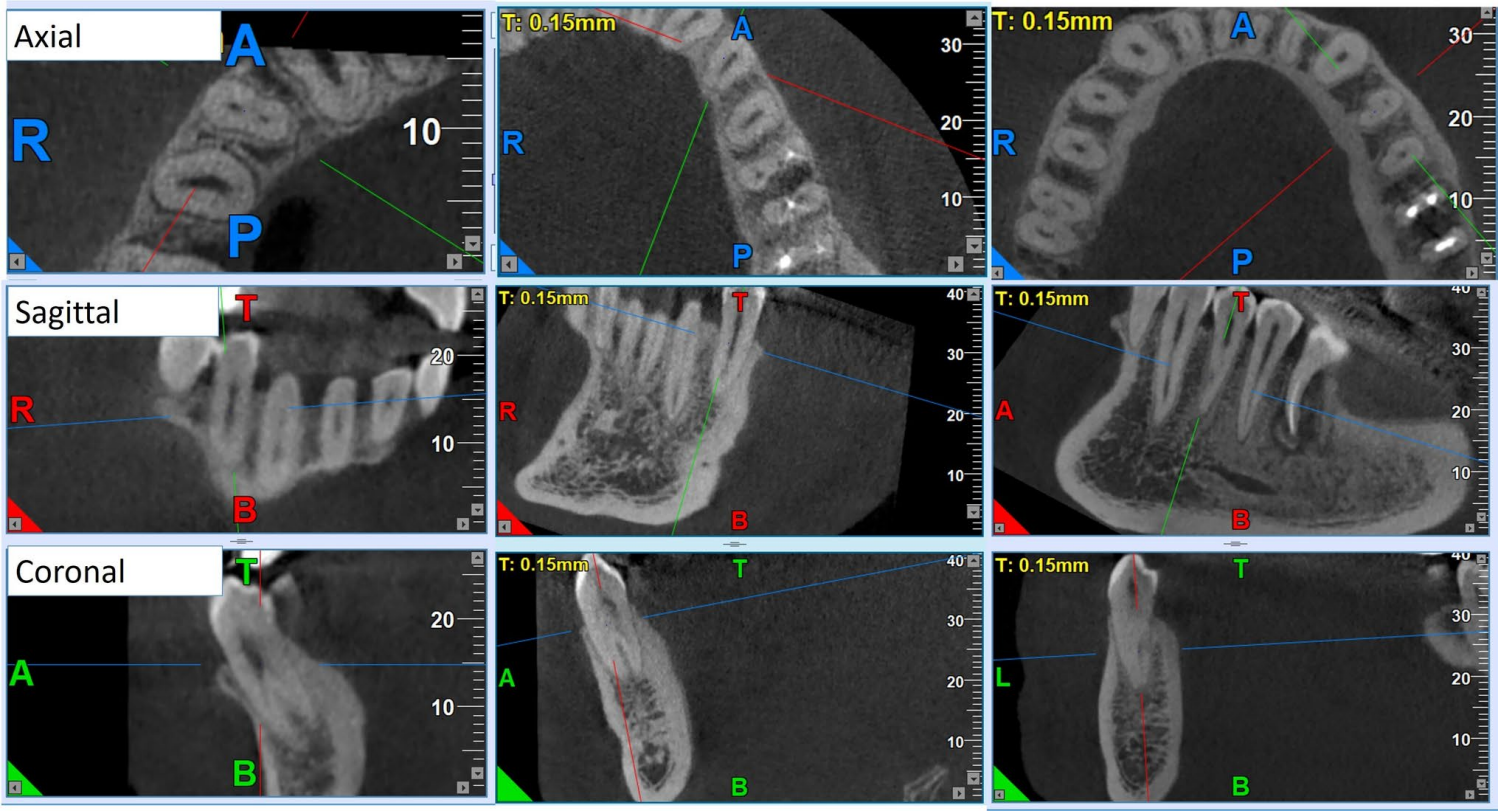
CBCT images of multirooted mandibular first premolars in axial, sagittal, and coronal views
